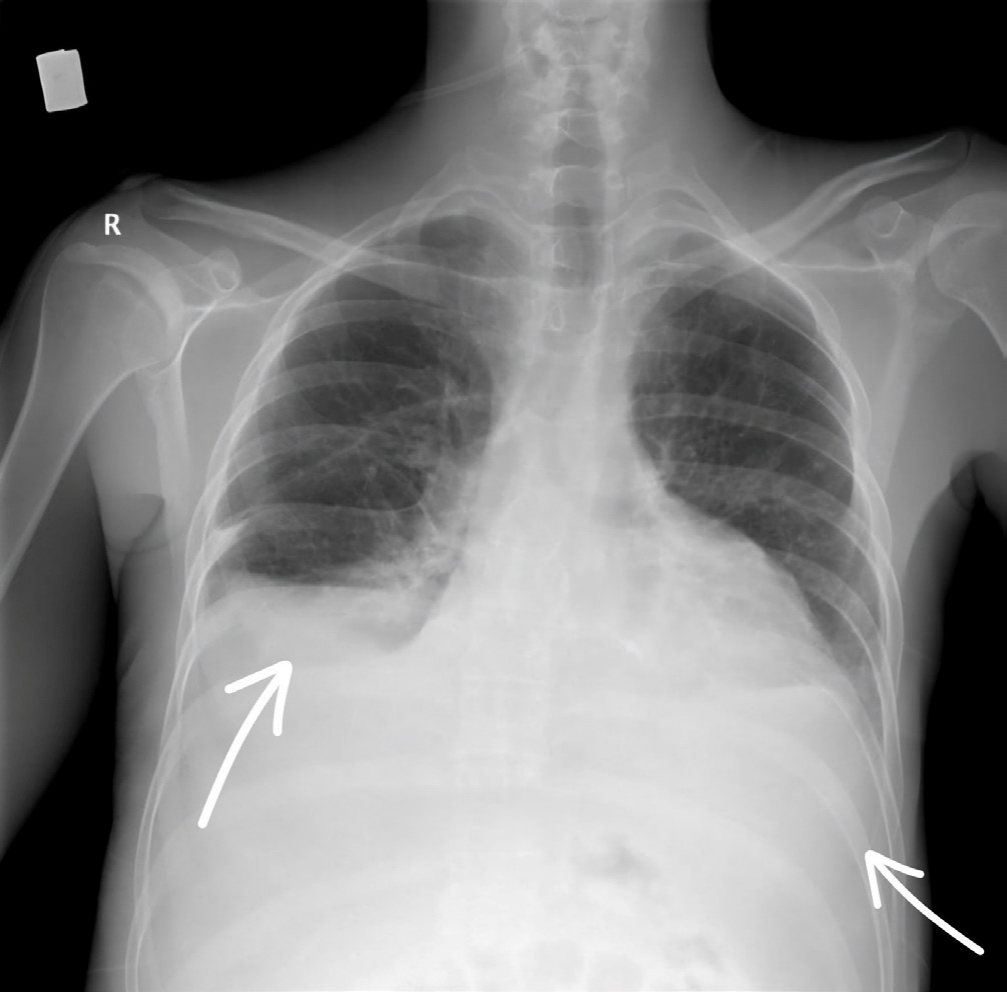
Chest X-ray after bilateral thoracentesis – no condensation foci, no signs of pneumothorax
